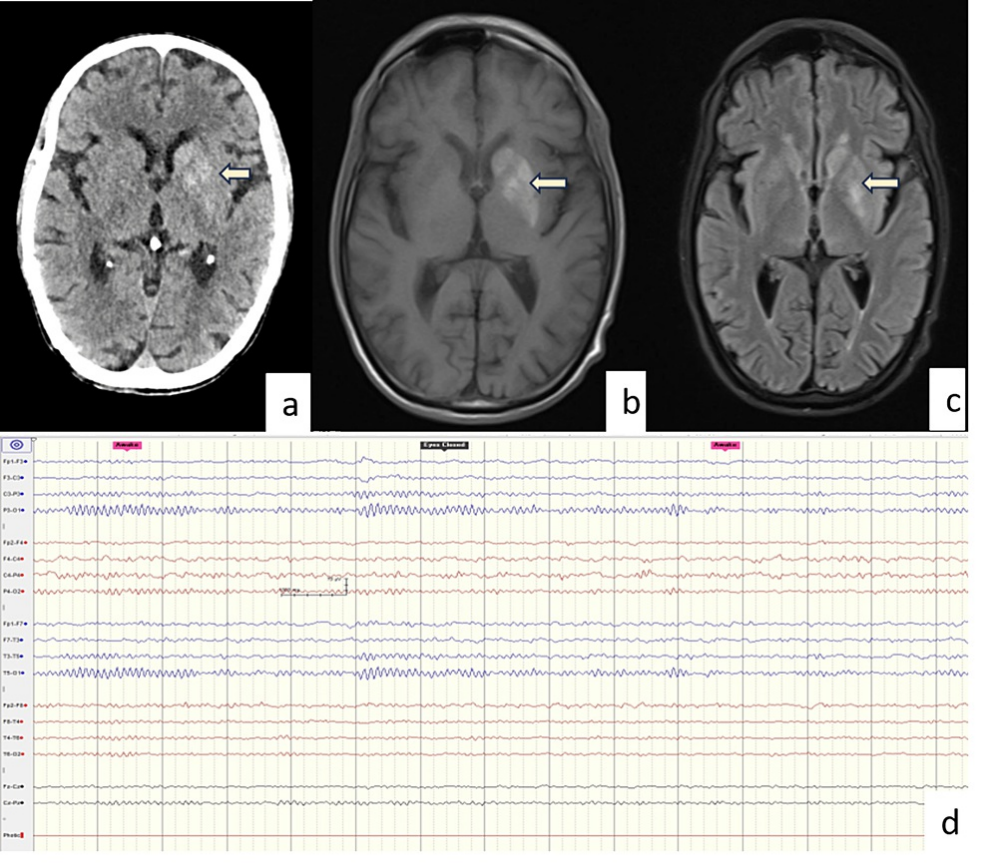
Neuroimaging (a) Brain CT of a 55-year-old male demonstrating hyperdensity (arrow) in the caudate and lentiform nucleus. MRI (axial) brain of the same patient showing hyperintensity (arrow) on T1W (b) as well as on T2W (c) imaging involving the caudate and lentiform nucleus. (d) Normal EEG of the same patient after the resolution of abnormal limb movement.
